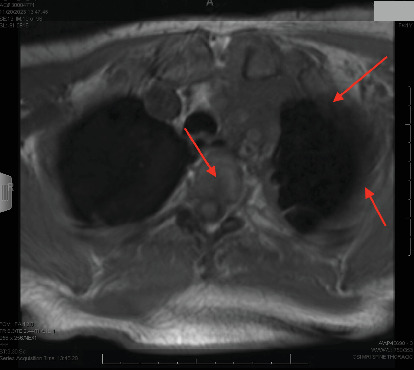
MRI of the thorax (axial). Extensive left-sided pleural thickening and spinal metastases can be seen.
